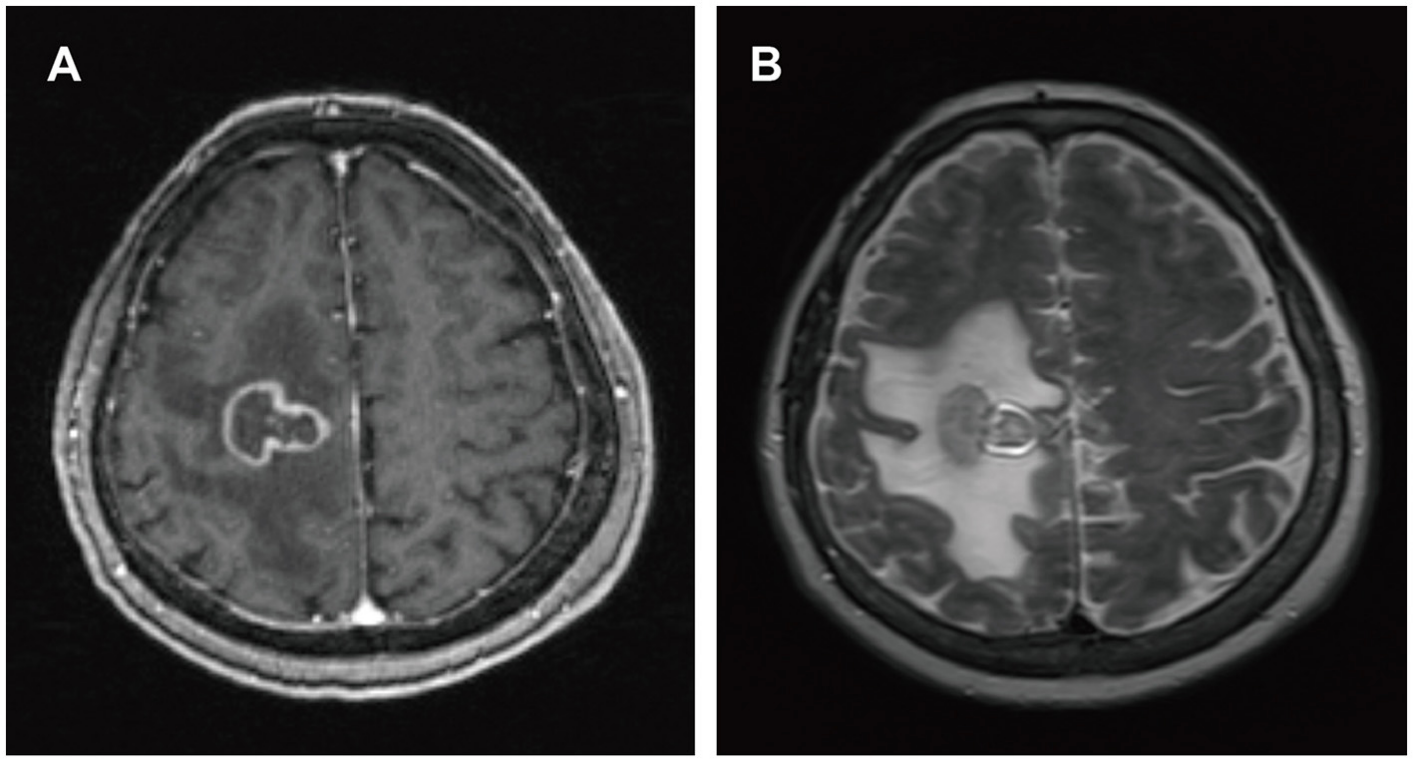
MRI findings of patient 1. Irregular ring enhancement lesion in the right frontal lobe on T1-weighted images (A). Extensive perilesional edema in the right frontal lobe on T2-weighted images (B).
